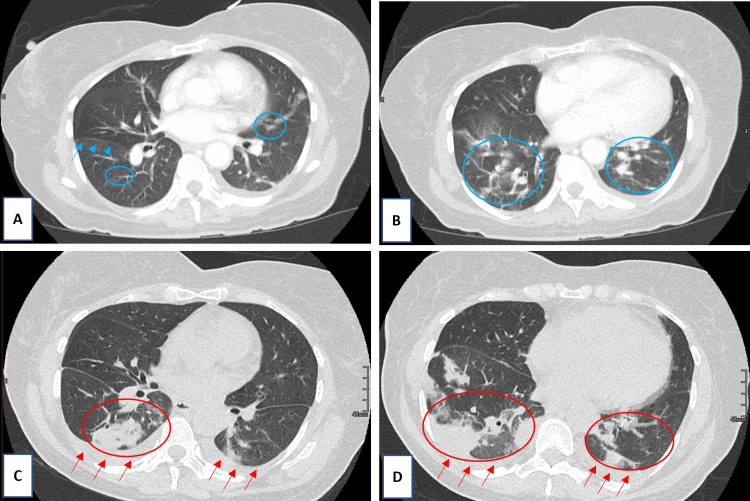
CT Chest serial axial images with A-B taken on initial evaluation (top row) and C-D taken on hospital day 4 with progression of multifocal lobar consolidation (bottom row). (A) Early right lower lobe perihilar ground-glass opacity (blue circles) and tracking along right middle lobe fissure (blue arrows); (B) Multifocal bilateral lower lobe consolidations (blue circles); (C) Formation of new right lower lobe consolidation (red circle) and progression of perihilar consolidation and bilateral pleural effusion (red arrows); (D) Interval worsening of bilateral lower lobe consolidation (red circles) and bilateral pleural effusion (red arrows).
